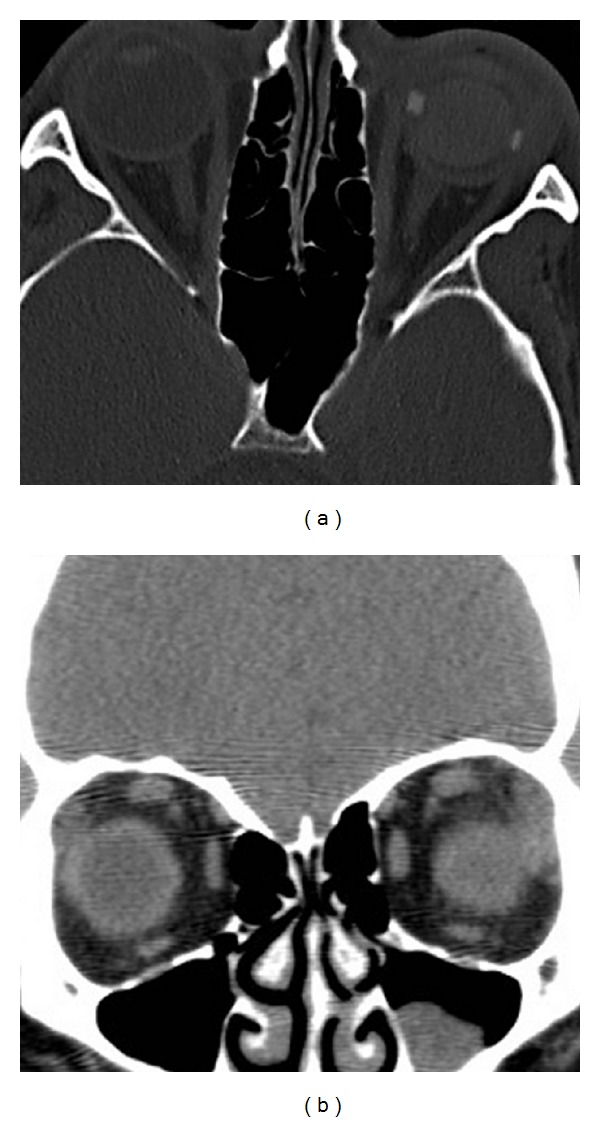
Orbital CT scan showing no residual intraocular foreign body.
